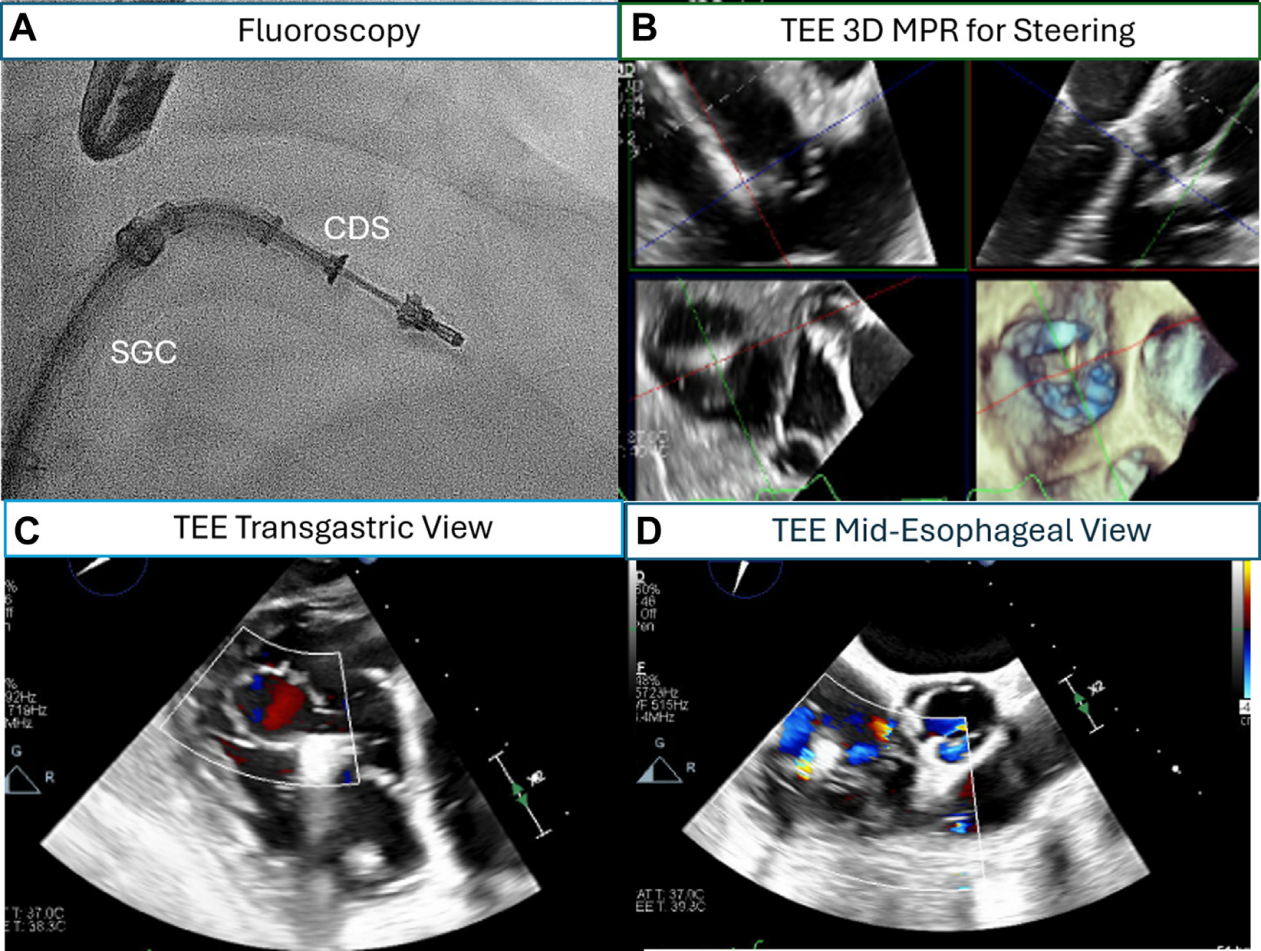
Intraprocedural Images of a Tricuspid-Transcatheter-Edge-to-Edge Repair Using the TriClip Device (A) Fluoroscopy of a TriClip Steerable Guide Catheter (SGC) and Clip Deliver (CDS) System. (B) Transesophageal echocardiogram (TEE) 3-dimensional (3D) multiplanar reconstruction (MPR) to guide the steering of TriClip system. (C) TEE transgastric view to confirm the clip arm orientation and tissue bridge after clip closure. (D) TEE midesophageal view to assess final tricuspid regurgitation after clip implantation.
